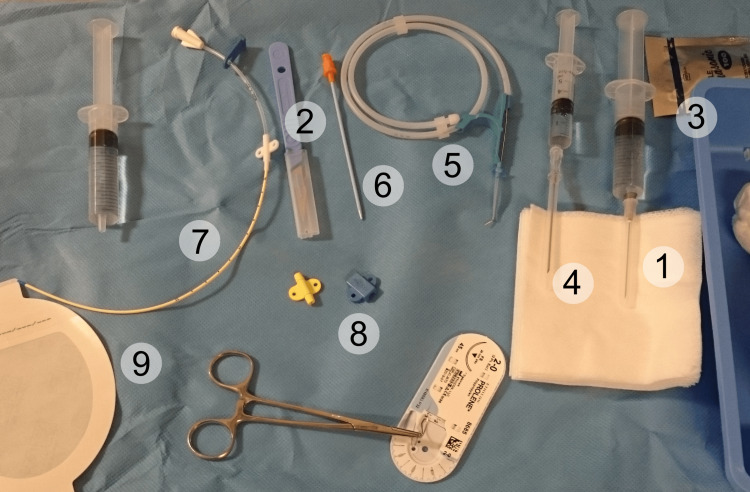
Standard central venous catheterization kit 1. Syringe with local anesthetic. 2. Scalpel. 3. Sterile gel for ultrasound probe. 4. Introducer needle. 5. Guidewire. 6. Tissue dilator. 7. Indwelling catheter. 8. Fasteners and suture. 9. Dressing. Image adapted from: Mikael Häggström. Central Venous Catheter set. Available from: https://openverse.org/image/8422e432-edea-42c3-9c4c-006e51ba32af?q=central+venous&p=11. Licensed under CC0 1.0
